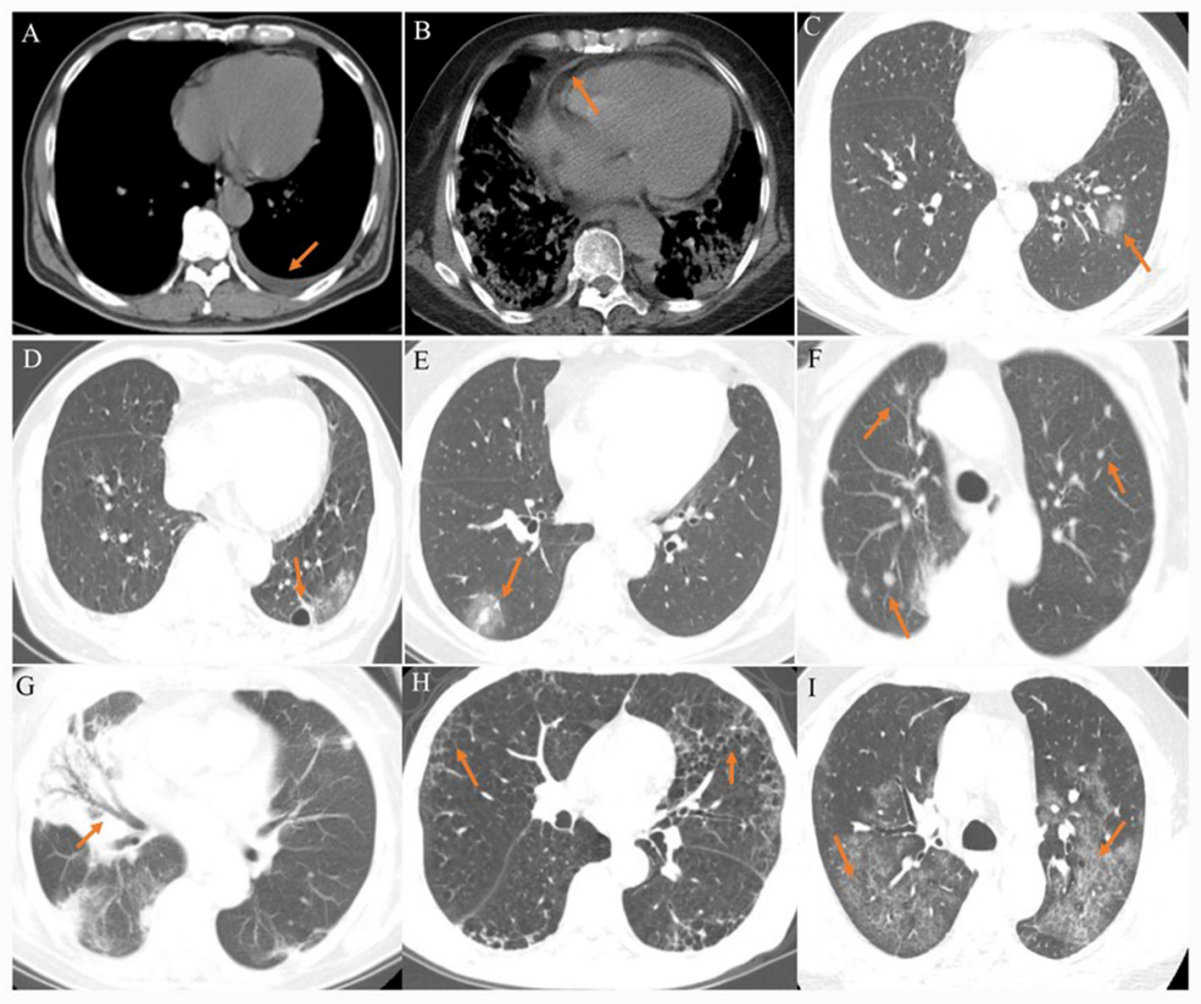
(A) A 40–45-year-old patient presenting fever for 5 days. CT scan shows left pleural effusion. (B) A 70–80-year-old patient presenting fever and shortness of breath for 10 days. CT scan shows pericardial effusion. (C) A 20–40-year-old patient presenting fever for 3 days. CT scan shows a ground-glass opacity in the left lower lobe. (D) A 40–45-year-old patient presenting fever with headache for 4 days. CT scan shows an air bubble sign in the left lower lobe. (E) A 30–50-year-old patient confirmed presenting fever for 4 days. CT scan shows a halo sign in the right lower lobe. (F) A 75–80-year-old patient presenting fever with cough for 9 days. CT scan shows bilateral multiple nodules. (G) A 40–45-year-old patient presenting fever for 8 days. CT scan shows air bronchogram in the middle lobe of right lung. (H) A 40–45-year-old patient presenting fever with cough for 5 days. CT scan shows bilateral reticular pattern. (I) A 40–45-year-old patient presenting fever with cough for 8 days. CT scan shows bilateral reticular pattern superimposed on the background of GGO, resembling the sign of crazy paving stones.
